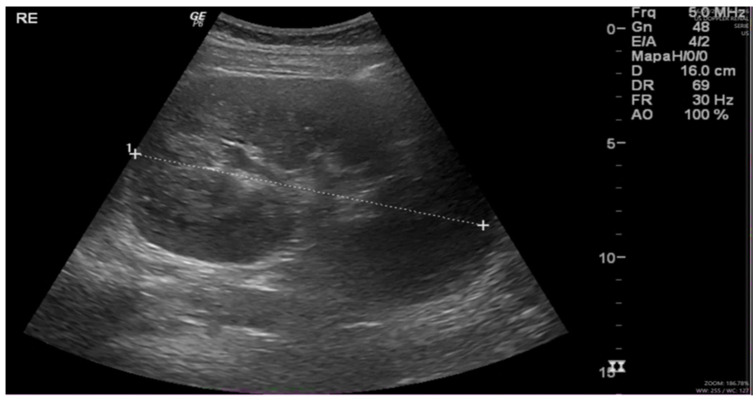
Renal ultrasound: kidneys in orthotopic position, with preserved contours and increased dimensions. Renal parenchyma with homogeneous thickness and echogenicity. Right kidney: 15.8 cm; parenchymal thickness: 2.2 cm. Left kidney: 16.0 cm; parenchymal thickness: 2.0 cm. No evidence of pelvicalyceal system dilation. Minimal amount of free fluid in the pelvis. (“1 to +” refers to the measurement of the left kidney’s size).
